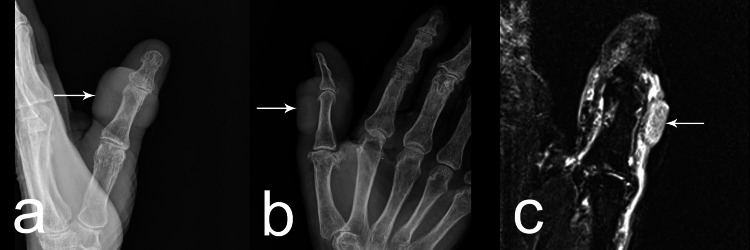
a,b: Roentgenography presented a clearly defined radiolucent ovoid formation in the thumb area (arrow) without involvement of bone structures (a – lateral view; b - anterior posterior view); c - Magnetic resonance imaging presented a semi-sleeve-shaped soft tissue heterogeneous formation (arrow) covering the dorso-lateral part of the finger's circumference.
